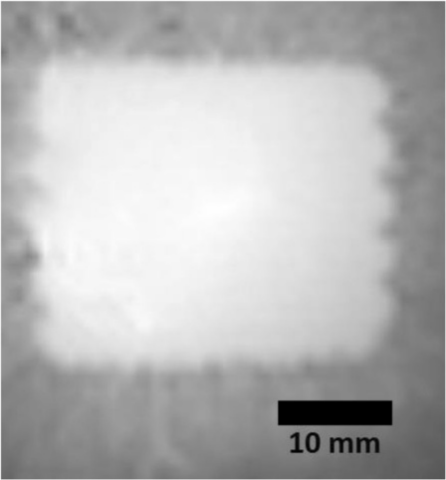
MRI of ex vivo sample following treatment. Scan plane orthogonal to transducer acoustic axis and positioned at geometric focus
